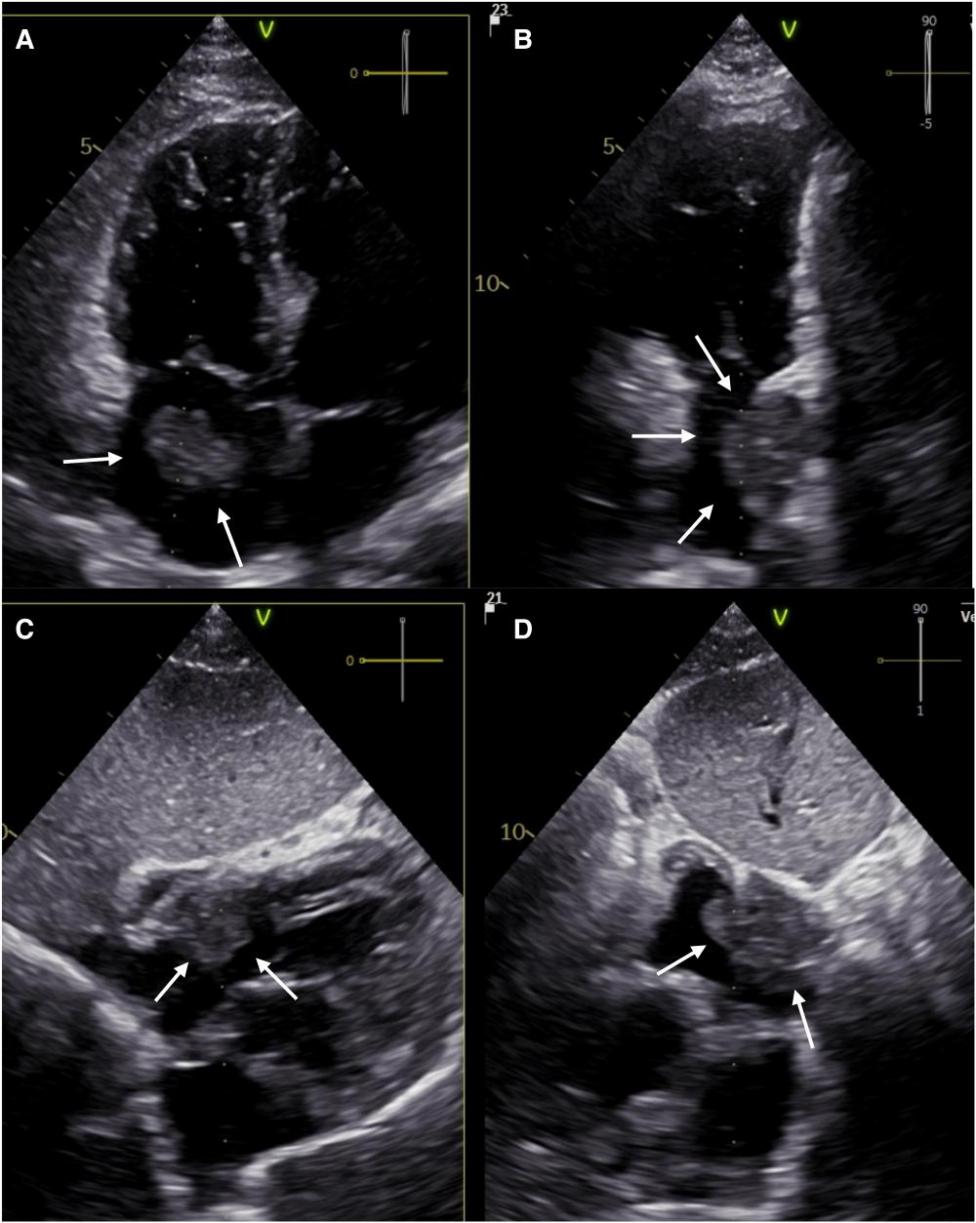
Transthoracic echocardiogram: (*A*) apical four-chamber view demonstrating right atrial mass (arrows), (*B*) corresponding orthogonal plane in the 3D echocardiogram, (*C*) subhypoid plane demonstrating right atrial mass (arrow), and (*D*) corresponding orthogonal plane in the 3D echocardiogram.
